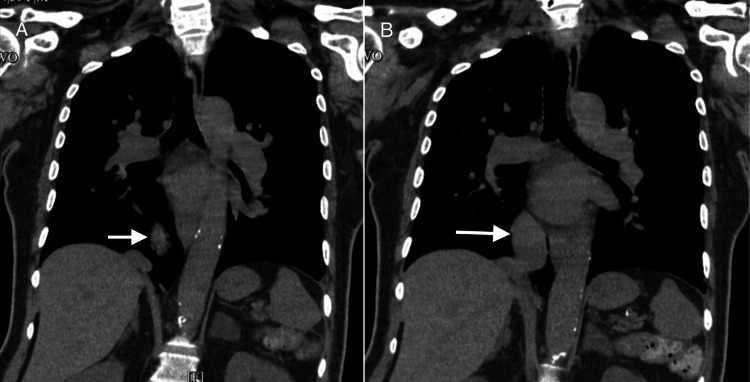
Chest computed tomography (CT) without contrast (coronal reconstruction at posterior level in A and more anteriorly in image B), demonstrating the scimitar vein, the aberrant connection of the pulmonary vein into the Inferior vena cava Chest CT at the level of the mid-to-lower thorax, capturing the mediastinal structures, including the lower portion of the trachea, bronchi, and adjacent lung parenchyma.
